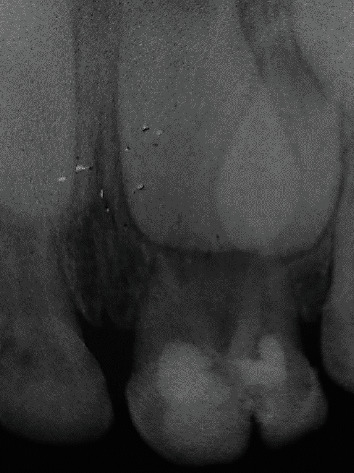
Periapical radiograph of the geminated tooth 61 and its contiguous tooth 62 at the 9-month follow-up visit: the presence of sealed coronal restoration of the two primary teeth with no pathological signs.
